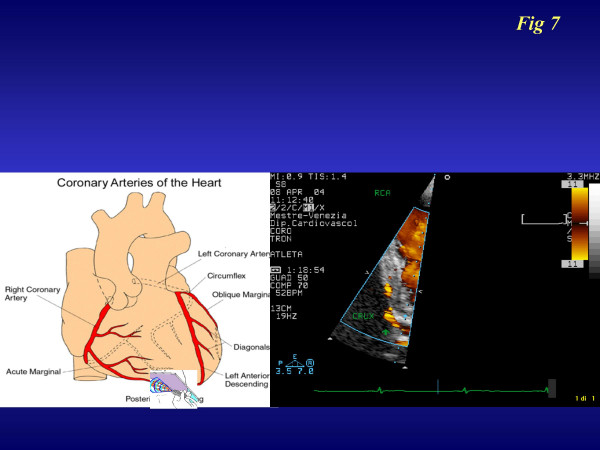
Imaging and projections for transthoracic imaging of right coronary and posterior descending coronary arteries.
